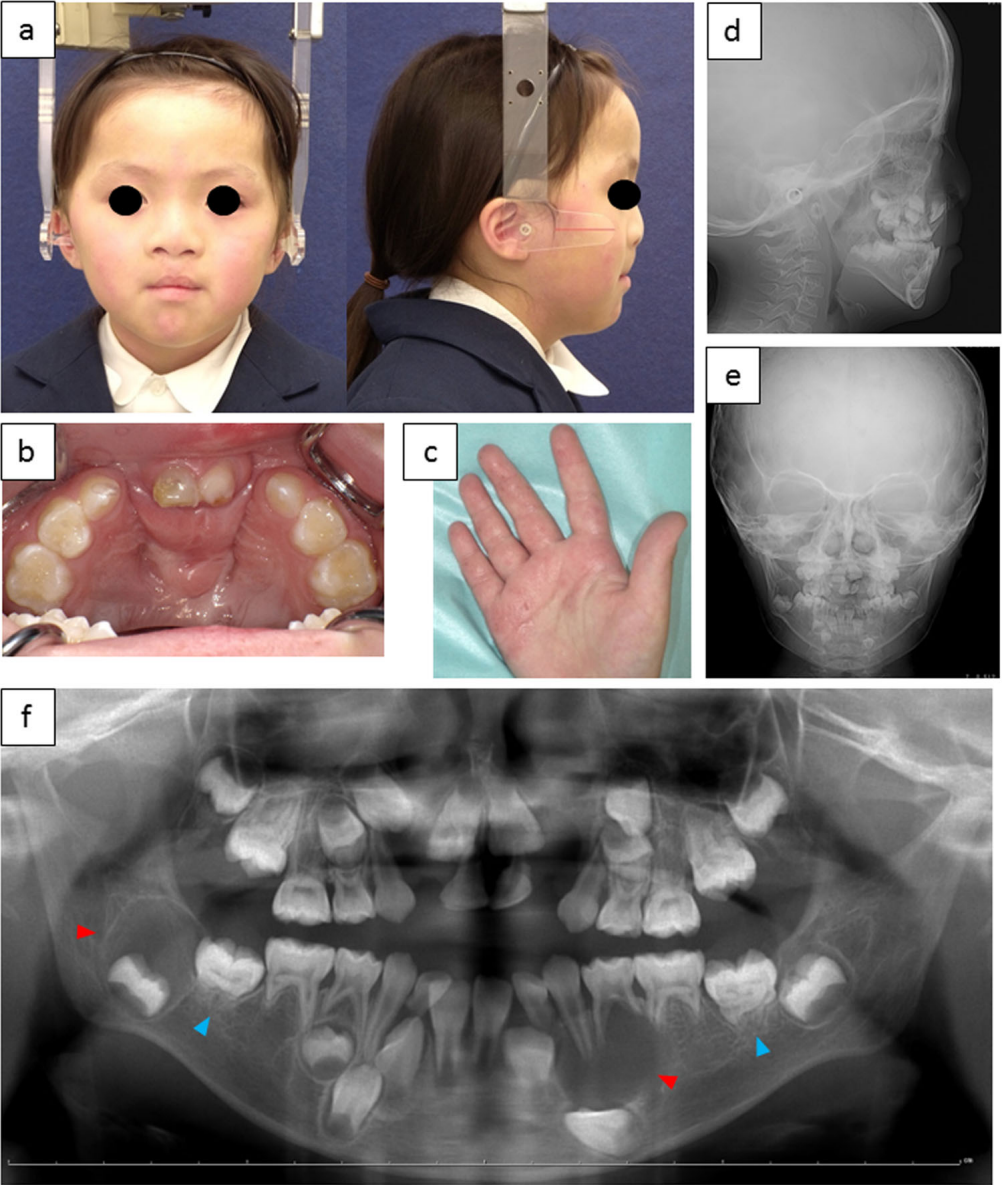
Clinical features of the present BCNS patient. a Facial photographs. b Cleft lip and palate. c Palmar pits. d, e Cephalometric radiograph. f Panoramic radiograph. Red arrows show multiple cystic radiolucent areas, and blue arrows show root hypoplasia
